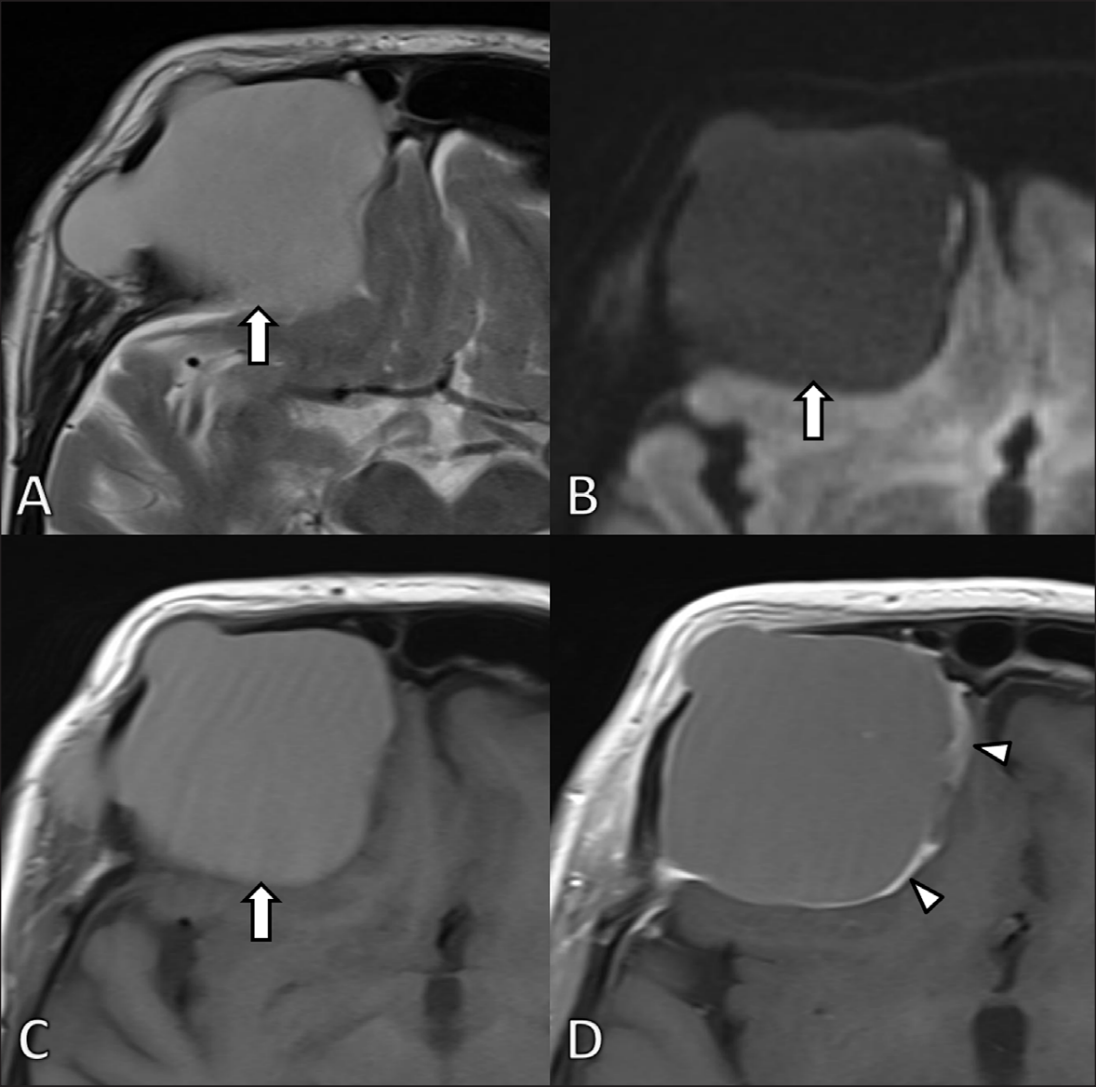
MRI at first admission. A. Axial T2-WI. B. Diffusion weighted image (b1000) and C. T1-WI before and D. after gadolinium contrast administration confirm the presence of a well-defined expansile mass (arrow) at the frontal bone. The signal is homogenously hyperintense on T2 and T1-WI images in keeping with high protein content. The lesion did not demonstrate restricted diffusion. After administration of gadolinium contrast the lesion shows subtle peripheral contrast enhancement (arrowheads). The lesion exerts mass effect on the frontal lobe.
